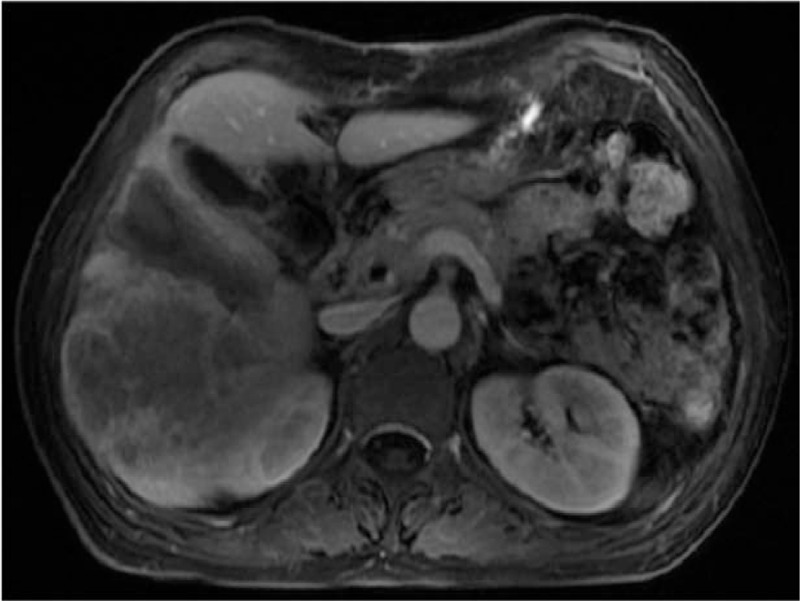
Magnetic resonance imaging scan shows coagulative necrosis of the hepatic parenchyma created by radiofrequency ablation.
